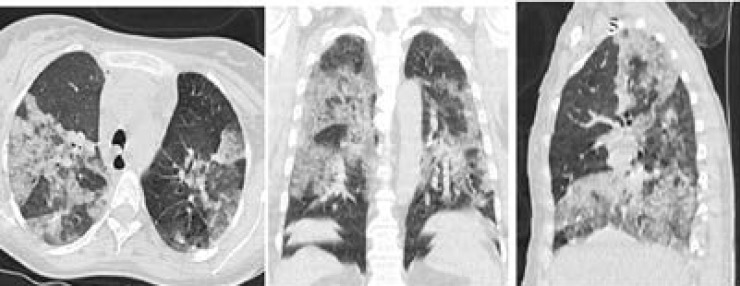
Initial thin-section non contrast Chest CT scan were performed on day of admission (from 2/4/2020): Axial, coronal and sagittal planes show multiple ground-glass opacities and a crazy paving pattern in bilateral lung.
